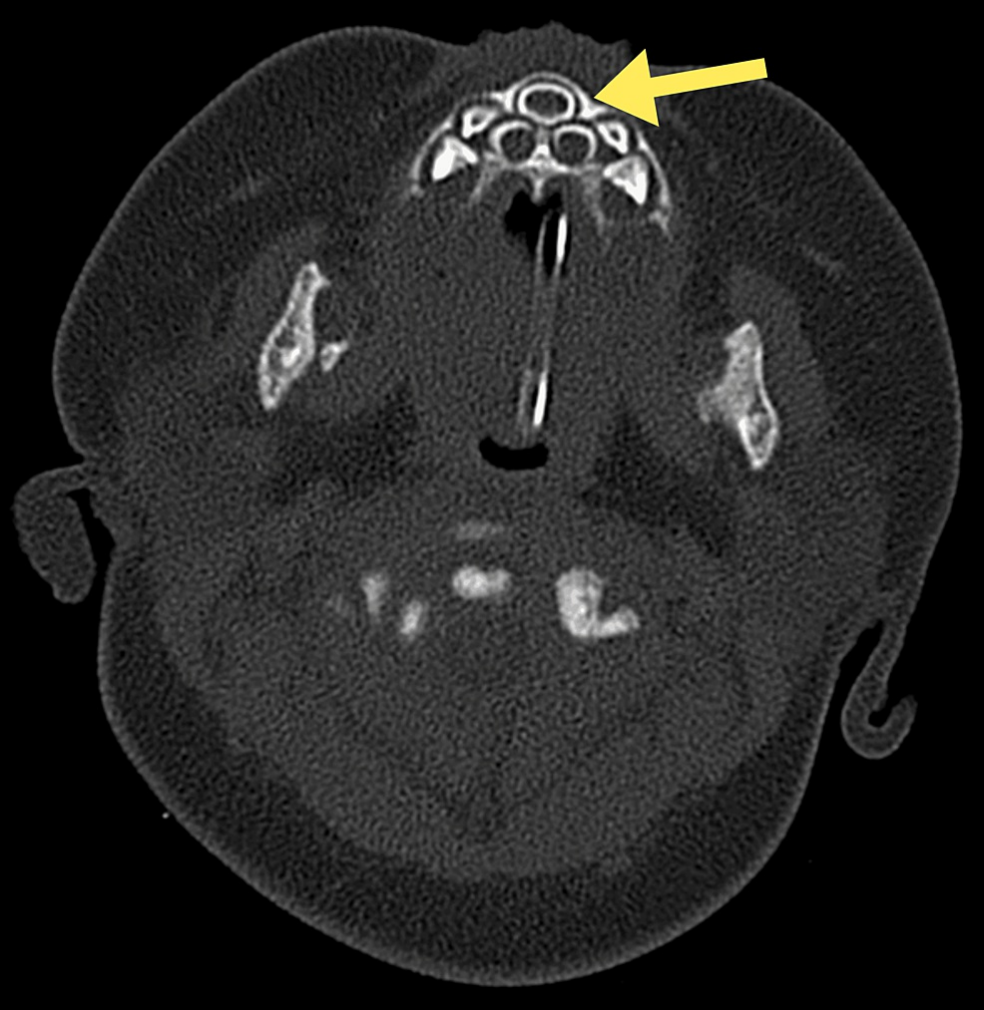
CT image shows SMMCI (yellow arrow). SMMCI, single median maxillary central incisor
